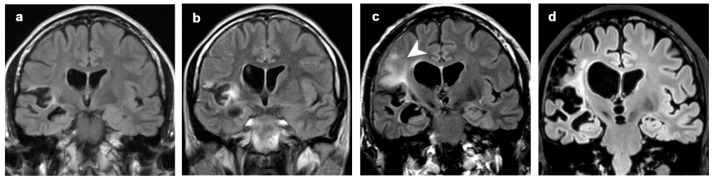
Patient 8, hyperintense signal intensities that preceded focal atrophy. Coronal FLAIR images at (a) 3 years, (b) 4 years, (c) 8 years and (d) 18 years from clinical onset. Focal atrophy limited to right perisylvian and insular region at the first MRI scan was followed by hyperintense alteration both of GM and WM in insula and frontal lobe (b,c, arrowhead). These alterations preceded worsening of the atrophic changes in the same areas.
